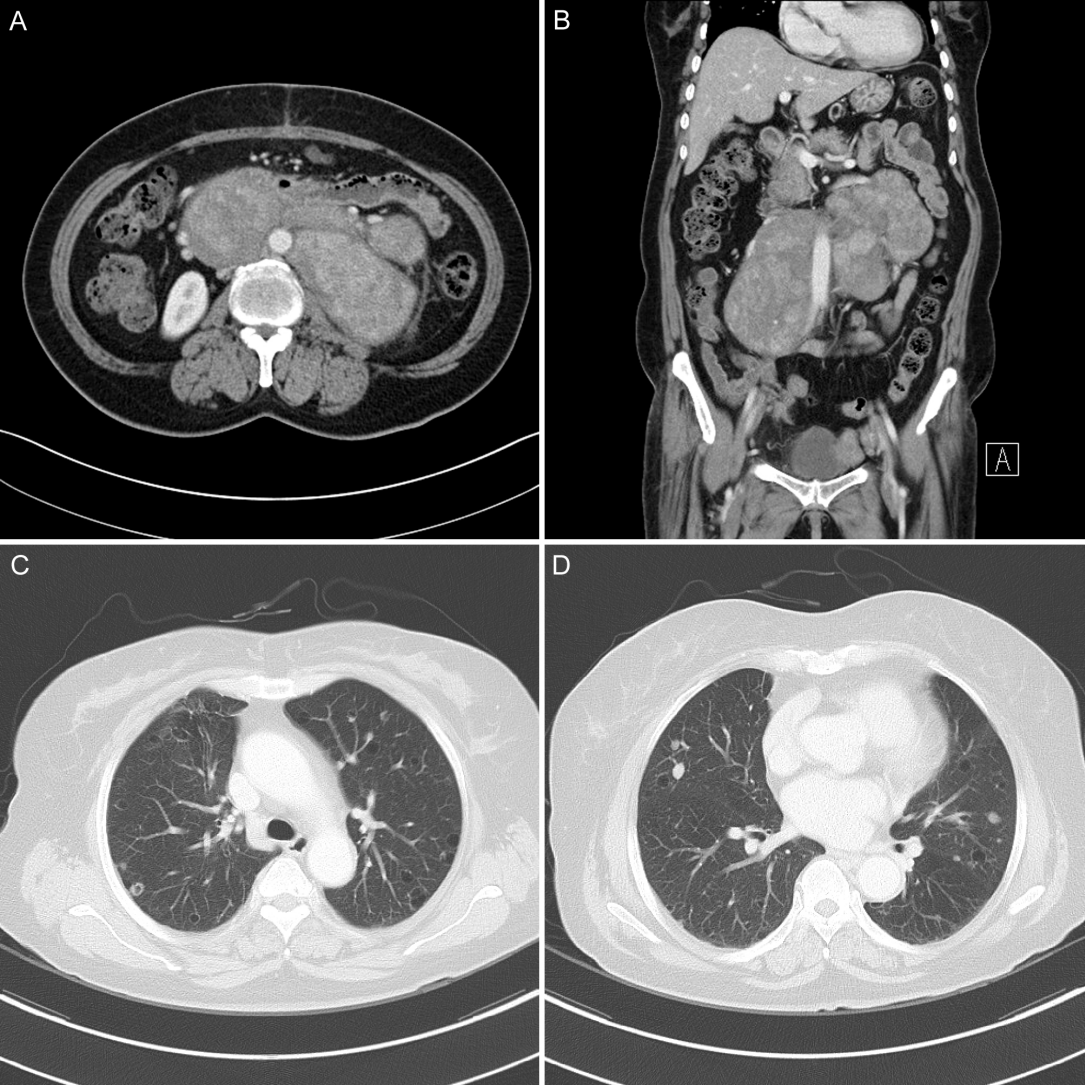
Computed tomography (CT) scan results. (a, b) Abdominal CT scan showing 8.8 × 16.7 cm sized multilobulated, well defined enhanced masses in retroperitoneal space. (c, d) Chest CT scan showing multiple tiny to small solid, cavitary metastatic nodules in both lungs.
